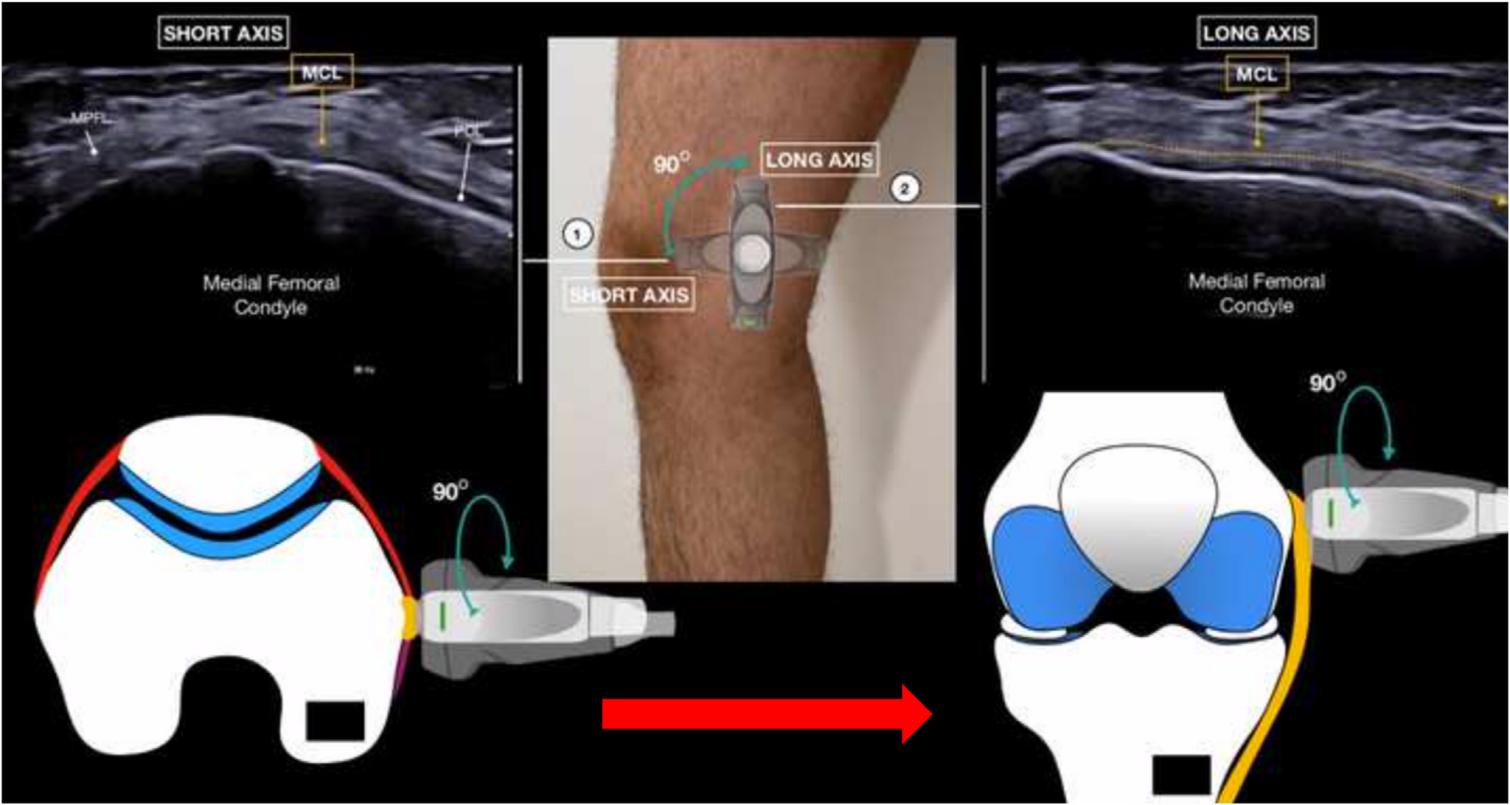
Once the MCL is identified on the ultrasound image, the probe should be held in place and rotated 90° such that it is in the long axis and follows the fibers of the MCL. (MCL, medial collateral ligament.)
